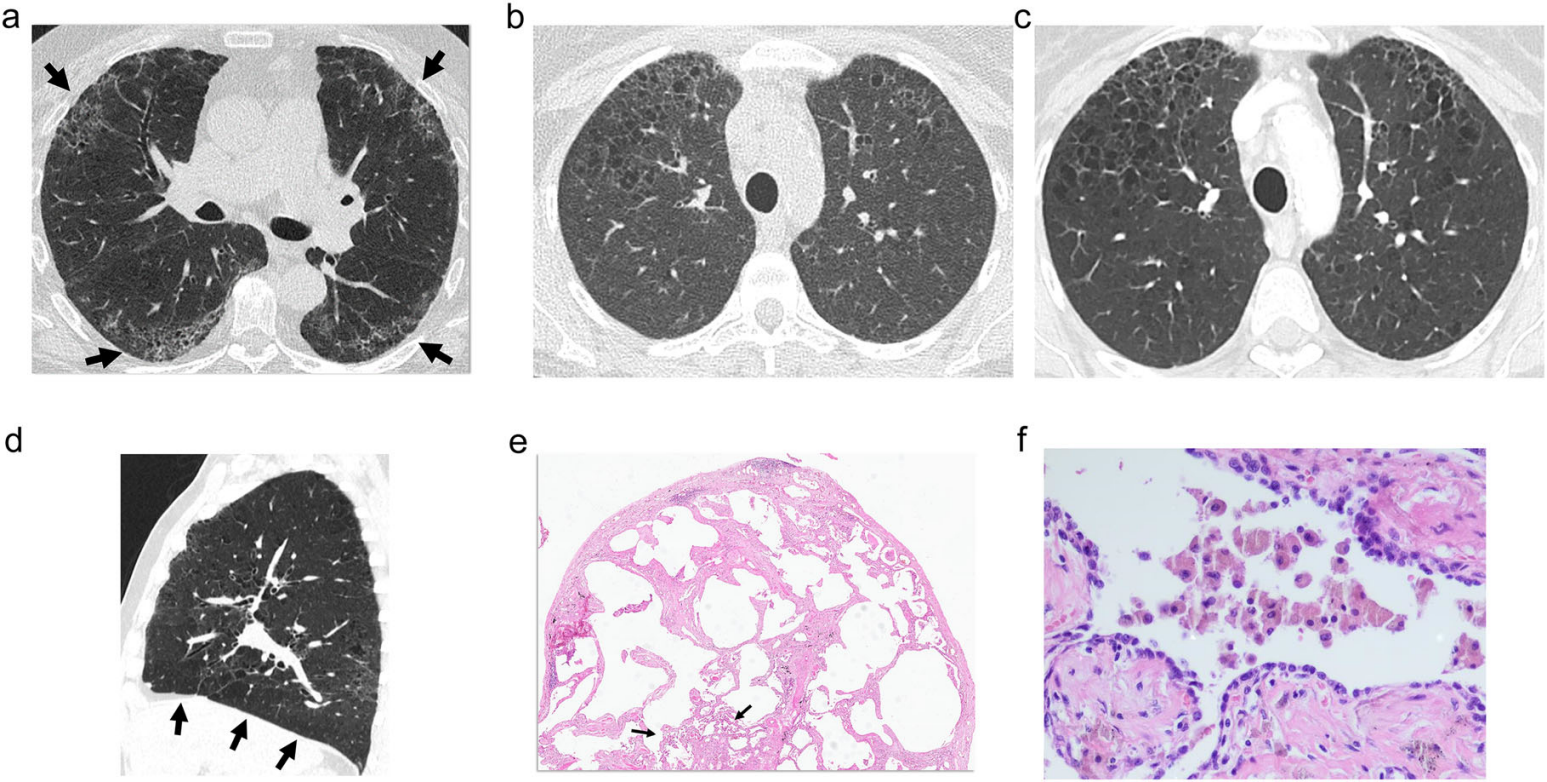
SRIF. Distribution of abnormalities in CT are shown in three different cases of pathologically confirmed SRIF: in a CT shows ground-glass, reticulation and some small cystic lesions in the periphery of the anterior aspects of the upper lobes and the posterior region of the upper segments of the lower lobes (arrows) mimicking “the four corners sign”; in b reticulation, emphysema and thin-walled cysts in the anterior upper lobes, that in a follow-up CT scan 15 years later (c) showed stability of findings without significant progression; and in d, sagittal reconstruction of the left lung showing how the findings are limited to the middle zones of the lung with sparing of the more basal diaphragmatic surface of the lungs (arrows). e At pathological examination corresponding to the upper segment of the left lower lobe of the patient in a, dense eosinophilic collagen thickening involves the alveolar septa and the subpleural area, together with some pigmented macrophages in the alveolar airspaces (arrows). f RB is shown with an accumulation of macrophages within the lumens of the respiratory bronchioles
